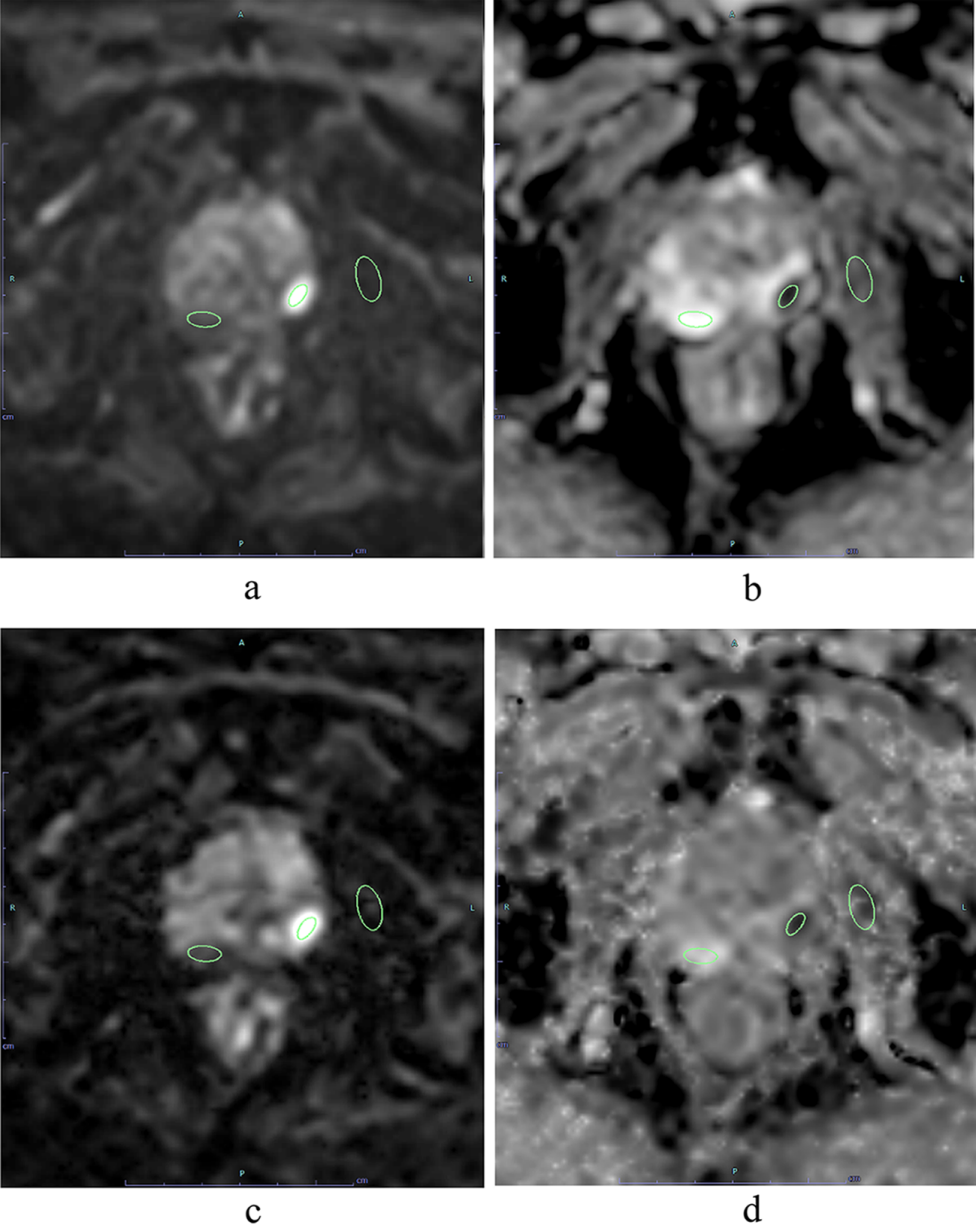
Region of interest (ROI) placement for signal intensity and ADC measurements of prostate cancer region in left peripheral zone and benign prostatic region in right peripheral zone and for signal intensity measurement of left internal obturator muscle region. The ROIs for PC region, benign prostatic region, and internal obturator muscle region in one of these four MR images was copy-and-pasted into the other three MR images [ssEPI DWI (a), ssEPI DWI ADC map (b), msEPI DWI (c), and msEPI DWI ADC map (d)].
